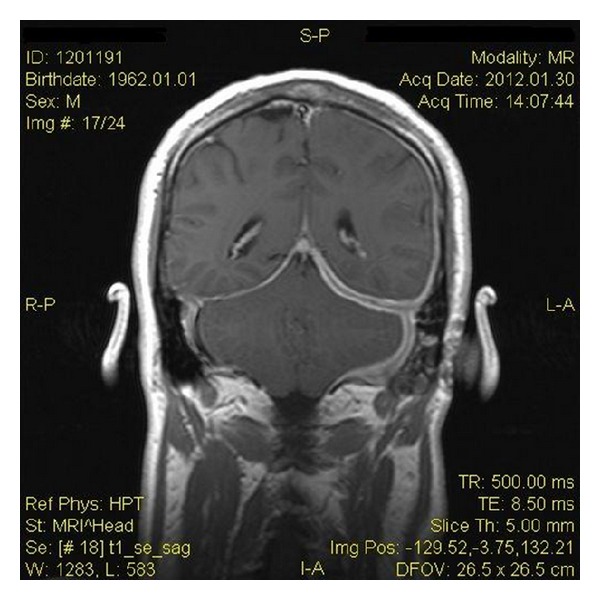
Gadolinium-enhanced coronal T1-weighted MRI scan showing pachymeningeal enhancement over the left temporal cerebral convexity, the left cerebellar convexity, and the left side of tentorium cerebelli. Also, there is left mastoiditis.
